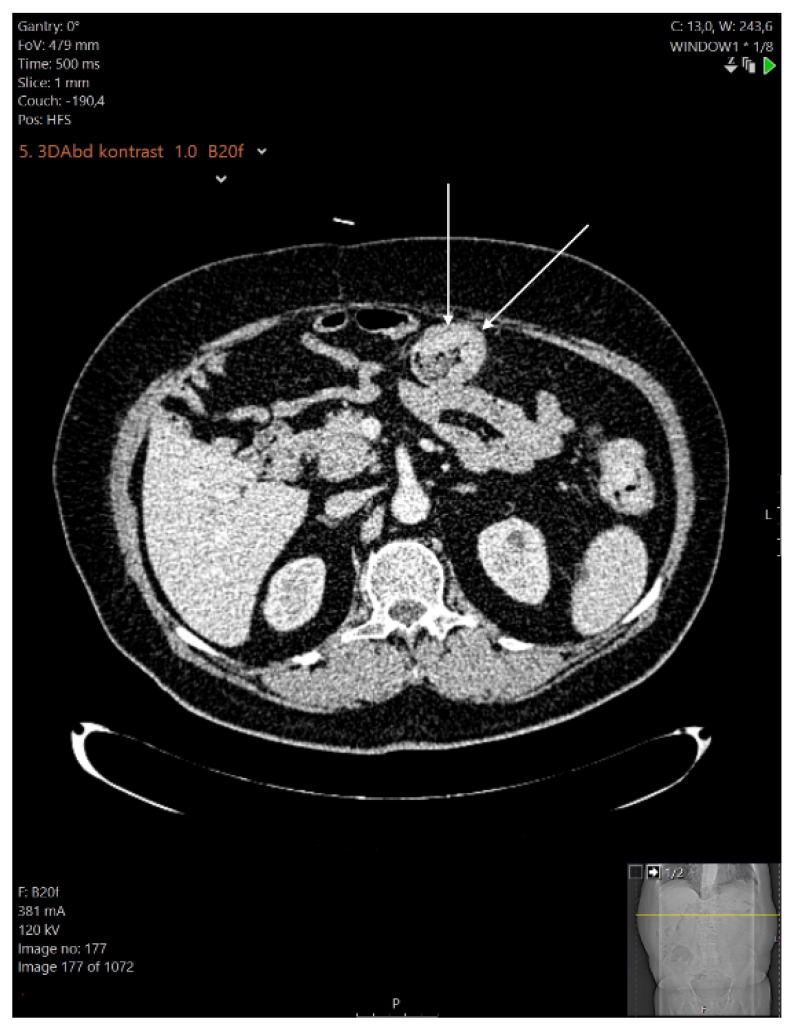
Abdominal multislice CT scan: colonic wall thickening with giant inflammatory polyps.
